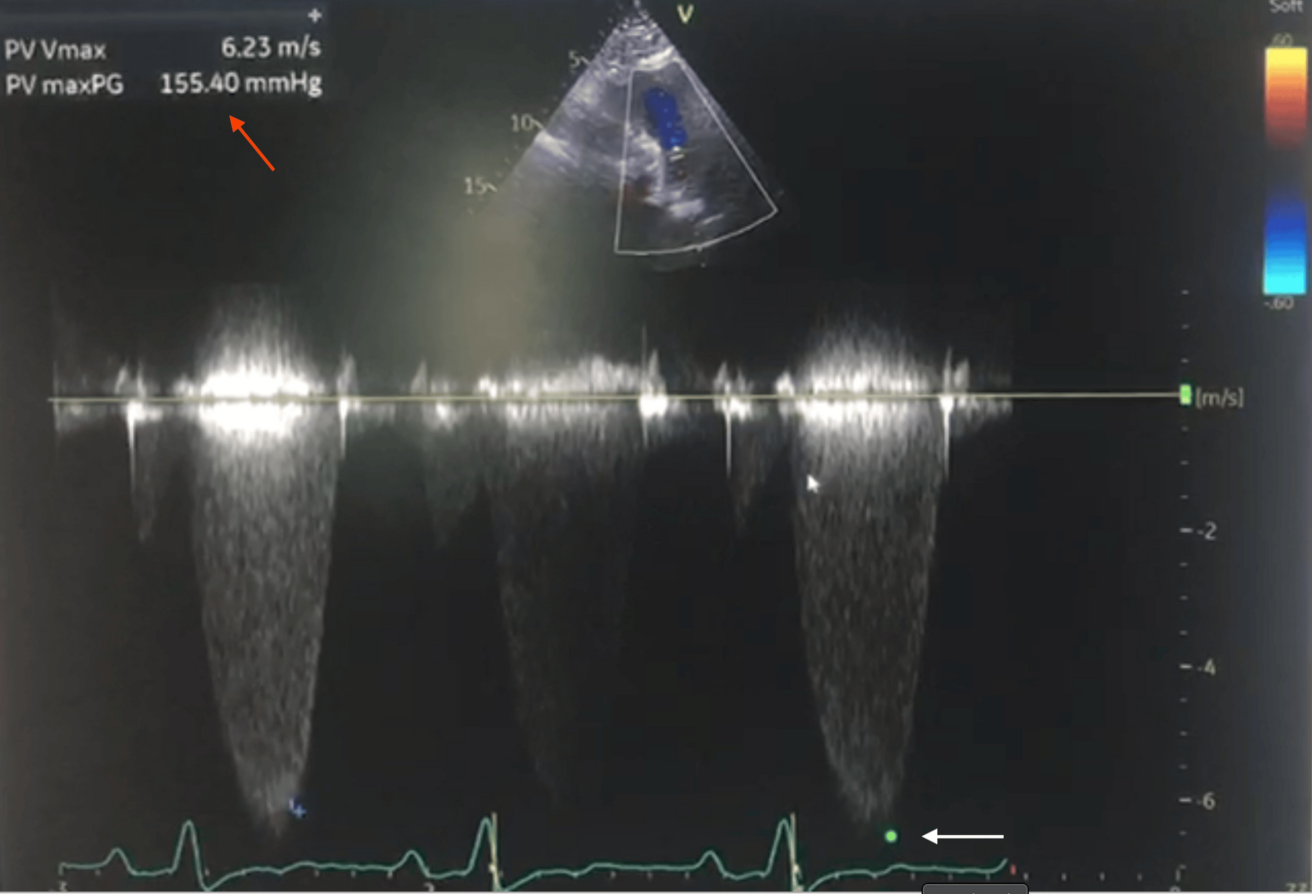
Trans-thoracic echocardiography with continuous-wave Doppler at the pulmonary valve Demonstrates markedly elevated velocity across the valve (white arrow). The calculated PV Vmax was 6.23 m/s with a peak pressure gradient of 155 mmHg (red arrow), consistent with severe valvular pulmonary stenosis. PG: pressure gradient; PV: pulmonary valve; PV Vmax: pulmonary valve maximal velocity.
